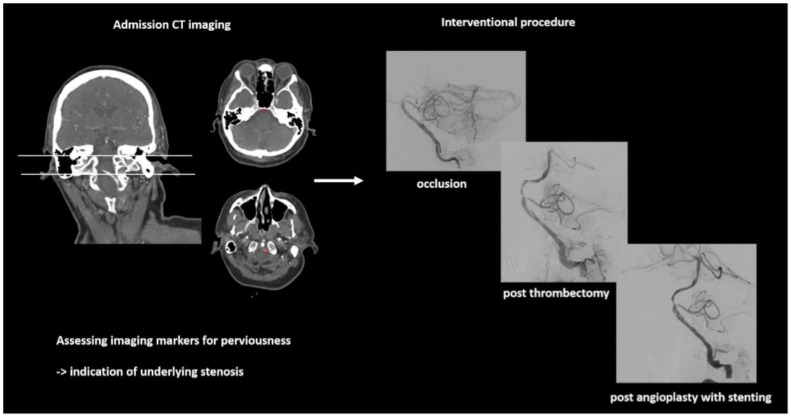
Example of a patient (male, 60 years) with acute occlusion of the basilar artery. In admission imaging, CT markers for perviousness were assessed. During endovascular therapy, underlying stenosis was detected after the first mechanical thrombectomy, resulting in recurring thrombus formation and the necessity for further thrombectomy maneuvers and final angioplasty with permanent stent placement.
